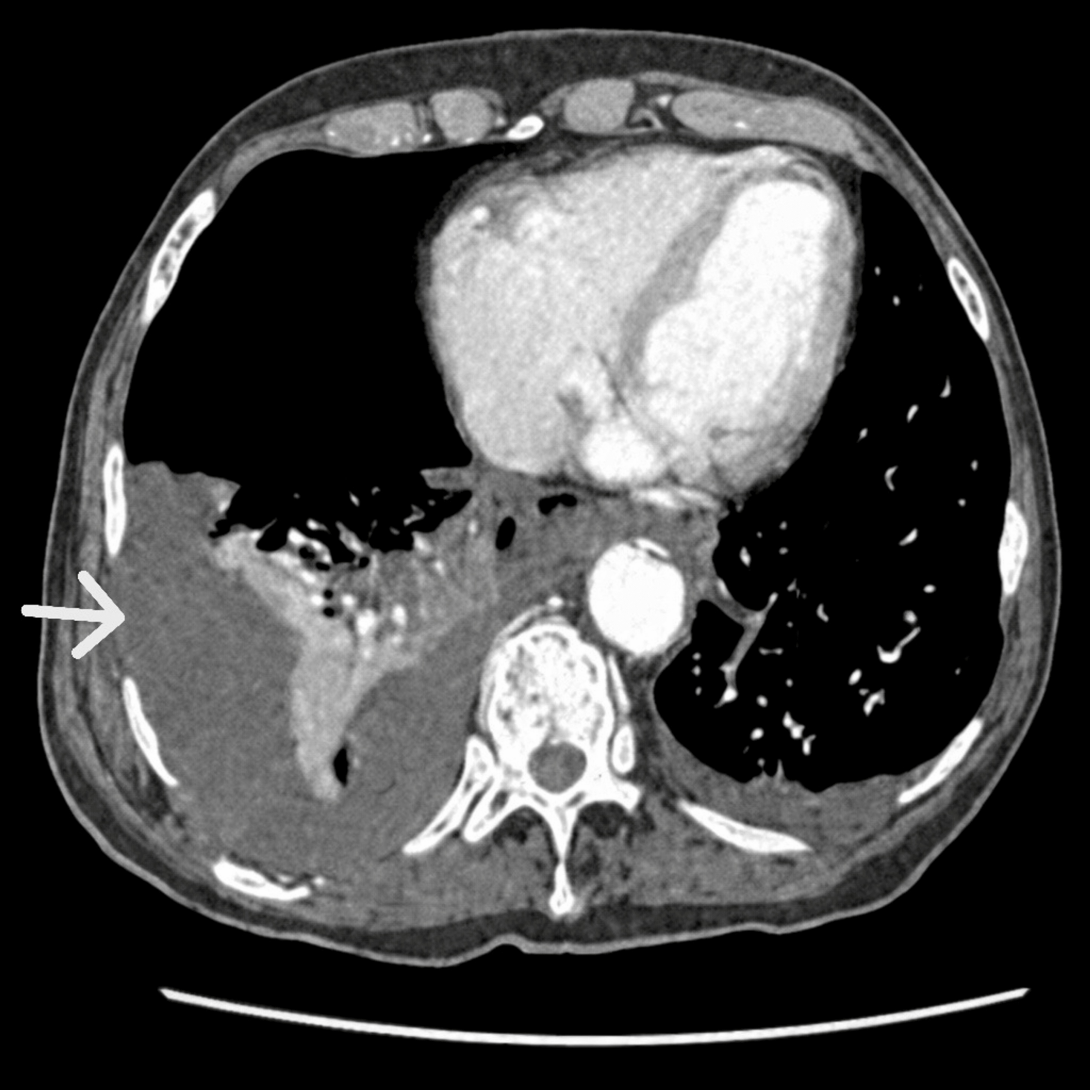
CT thorax (venous phase) shows a large right-sided hydropneumothorax with complete collapse of the right lung and mediastinal air posterior to the gastroesophageal junction (arrow), consistent with esophageal perforation.
